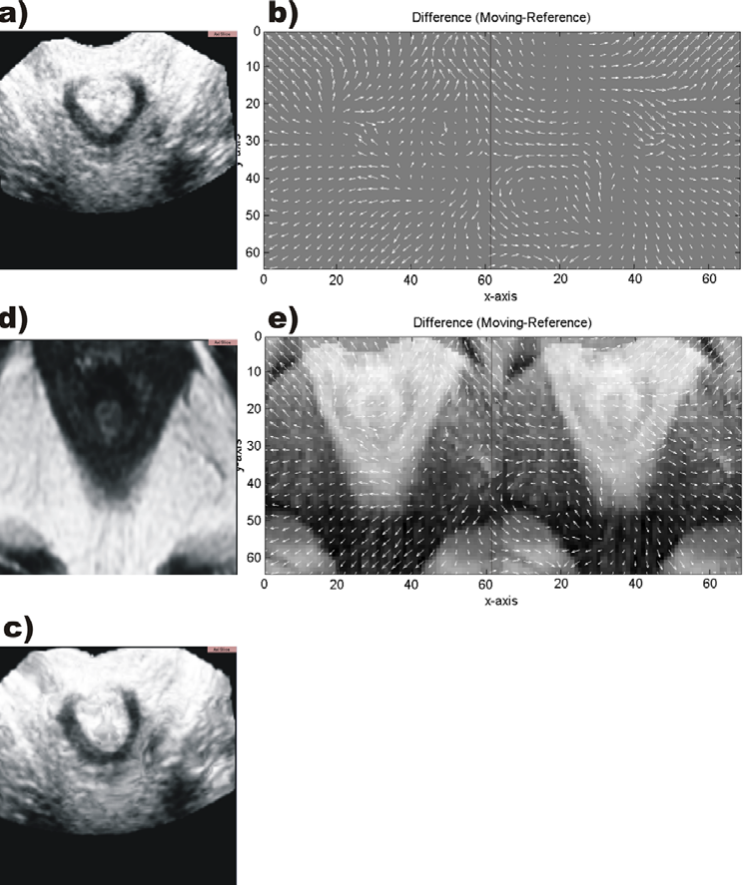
Registration and displacement field. Axial plane through non-rigid registration of the anal canal: Original data are shown in (a) and (d) – US and MR image, respectively. For simplification of visualization only the difference components in x-direction (b left) and in y-direction (b right) are shown. The difference in z-direction is ommited. (e) shows the difference image of (a) and (d) together with the difference components in x direction (e left) and in y direction (e right). (c) shows the registered image after the application of the displacement field.
